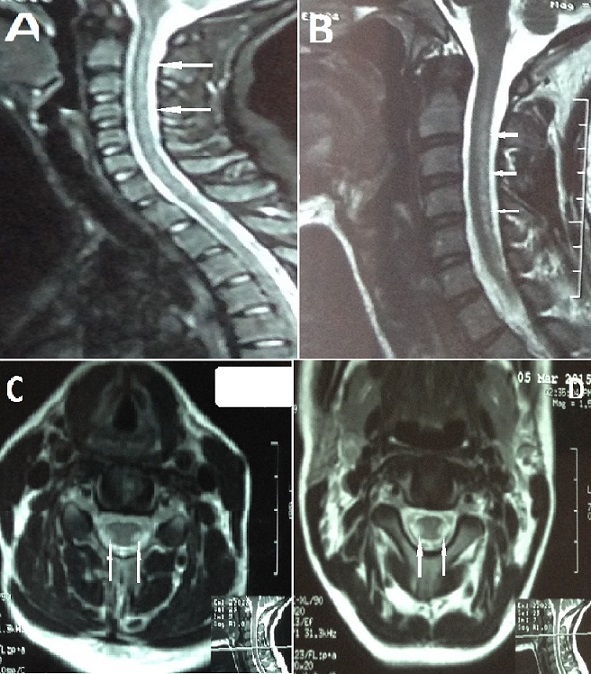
(A,B) MRI of the spinal cord, in sagittal T2 weighted image showing an increased signal intensity extended to the whole cervical spinal cord; (C,D) MRI of the spinal cord, in axial T2 weighted image, showing increased bilateral and symmetrical signal intensity of lateral and posterior funiculus.
